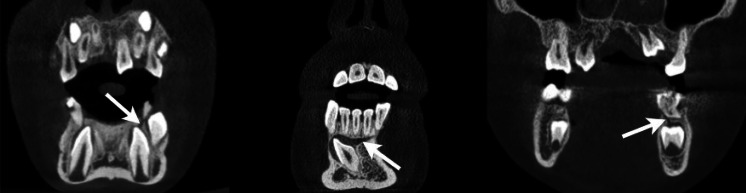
Coronal image on CBCT of the gubernacular canal (marked by arrow) in erupting teeth
